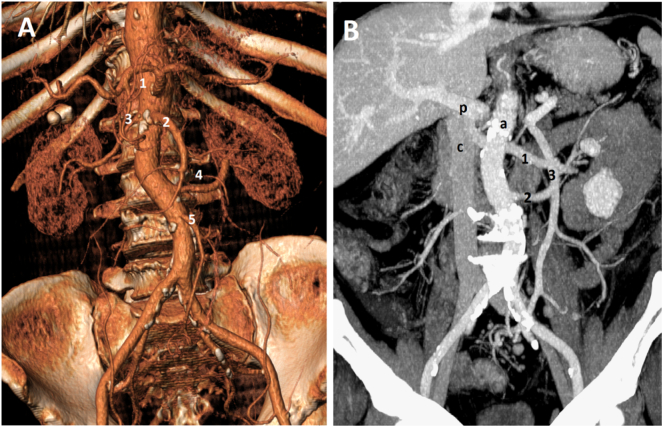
In the three-dimensional CT angiography (A) is highlighted the distal origin of the left renal artery (4) and the normal origin of the other vascular structures: (1) celiac trunk, (2) mesenteric superior artery, (3) right renal artery, (5) inferior mesenteric artery. The CT-scan (B) underlines the anomalous distance between the left renal vein (1) and left renal artery (2). P) portal vein; a) aorta; c) vena cava; 3) mesenteric inferior vein.
